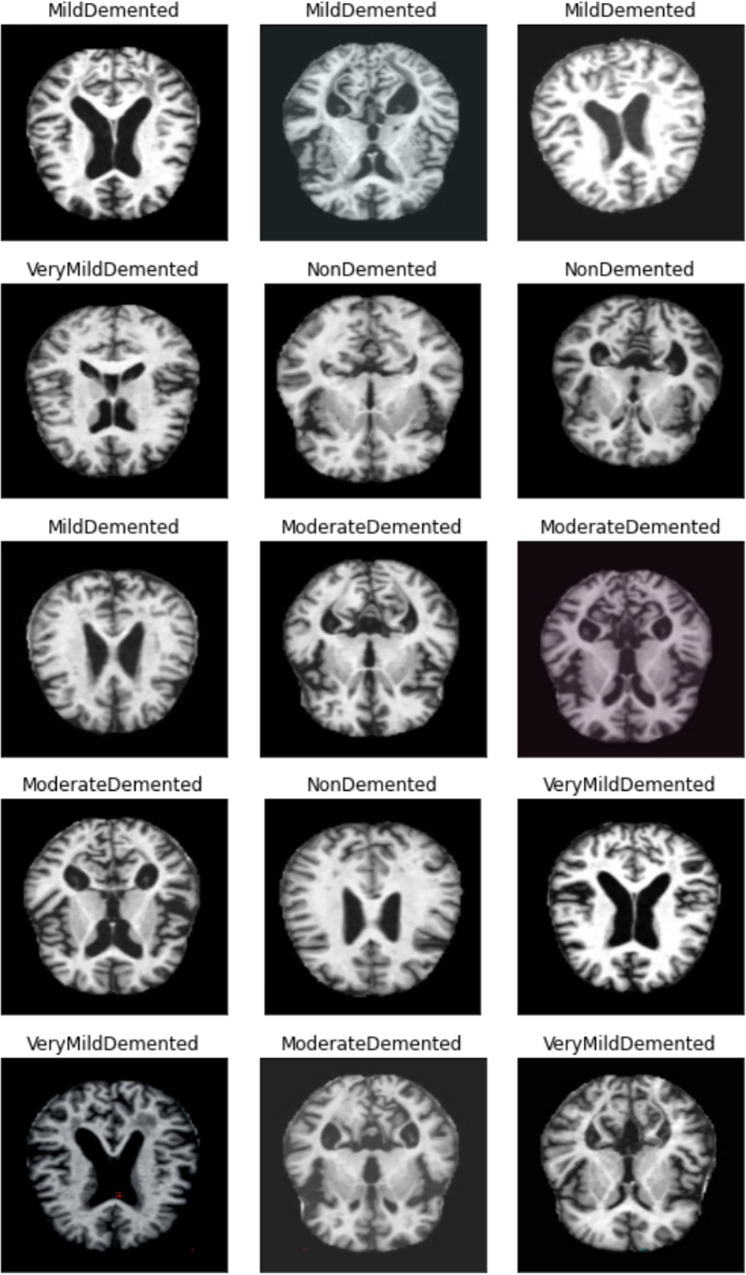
Sample images from the Alzheimer’s MRI brain dataset^33^ for each of the four classes.
